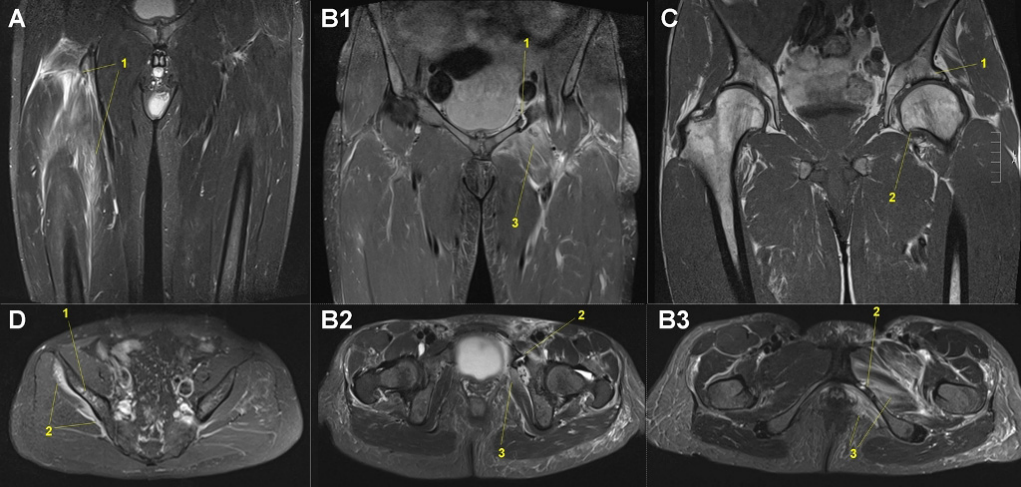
Mobile MRI of pelvis region and hip joints. A: male, 45-years-old, stage 8, 511 km (PDw TSE fs coronal): Massive edema and (peri-)myositis of right proximal quadriceps muscle (1). B: female, 46-years-old, stage 48, 3,290 km (PDw TSE fs, B1: coronal, B2,3: transversal): Stress fracture of left ventral pelvic ring (1: Ramus superior ossis pubis; 2: Ramus inferior ossis pubis) with perifocal soft tissue edema/inflammation (3). C: male, 41-years old, stage 11, 739 km (T1 TSE coronal): Arthrosis of the left hip (1: acetabular sclerosis, 2: deformation and osteophyte of the femoral head). D: male, 61-years-old, stage 38, 2601 km (PDw TSE fs transversal): Intraosseus edema in the right Ala ossis ileum (1) with massive peri-osseal inflammation of the gluteal muscle origin (2).
